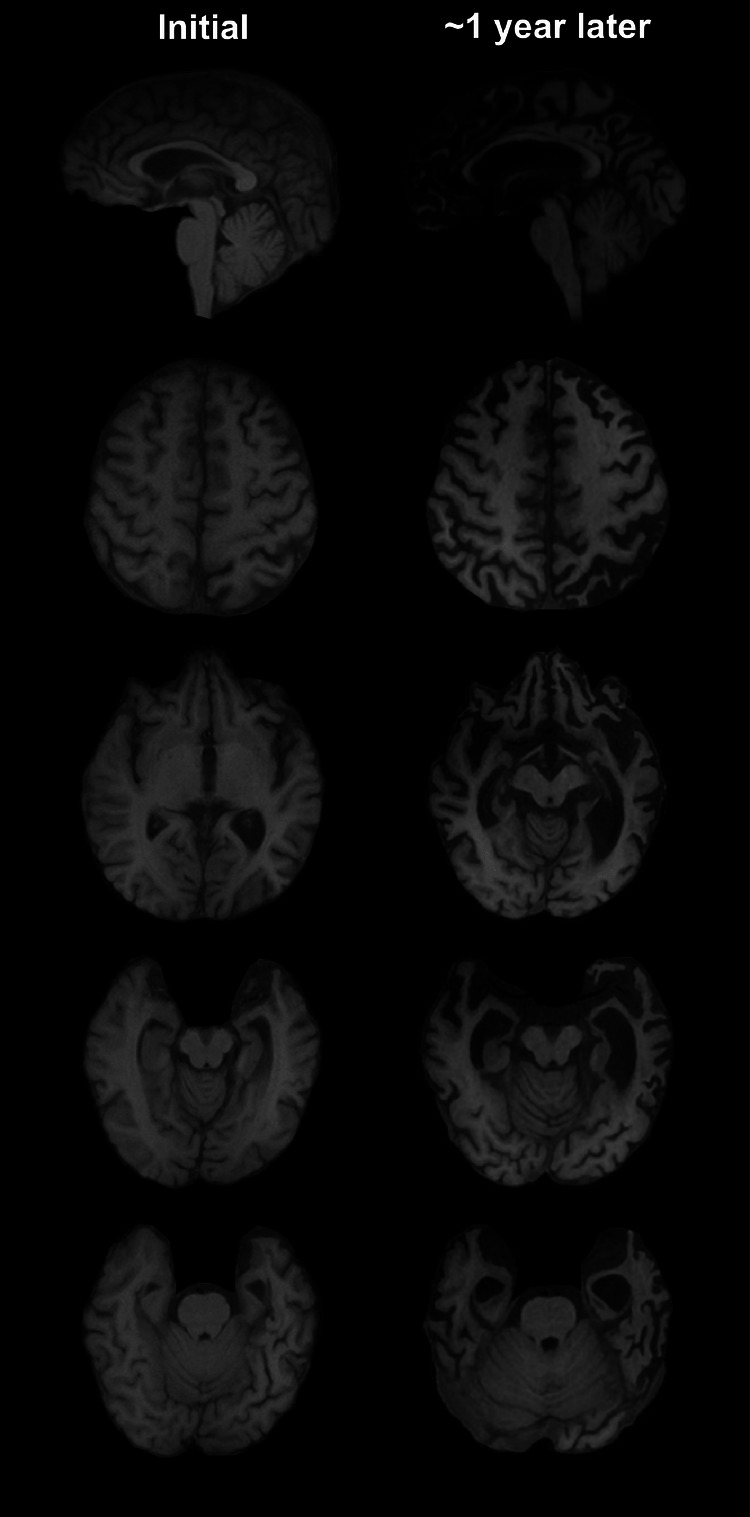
MRI reveals rapidly progressive brain atrophy. Outside brain imaging was compared to follow-up brain MRI (via a 3-T scanner). The initial MRI shows subtle left greater than right amygdala and mesial temporal atrophy. An MRI done approximately 1 year later shows progressive knife-edge atrophy now involving bilateral hemispheres, with left greater than right involvement.
